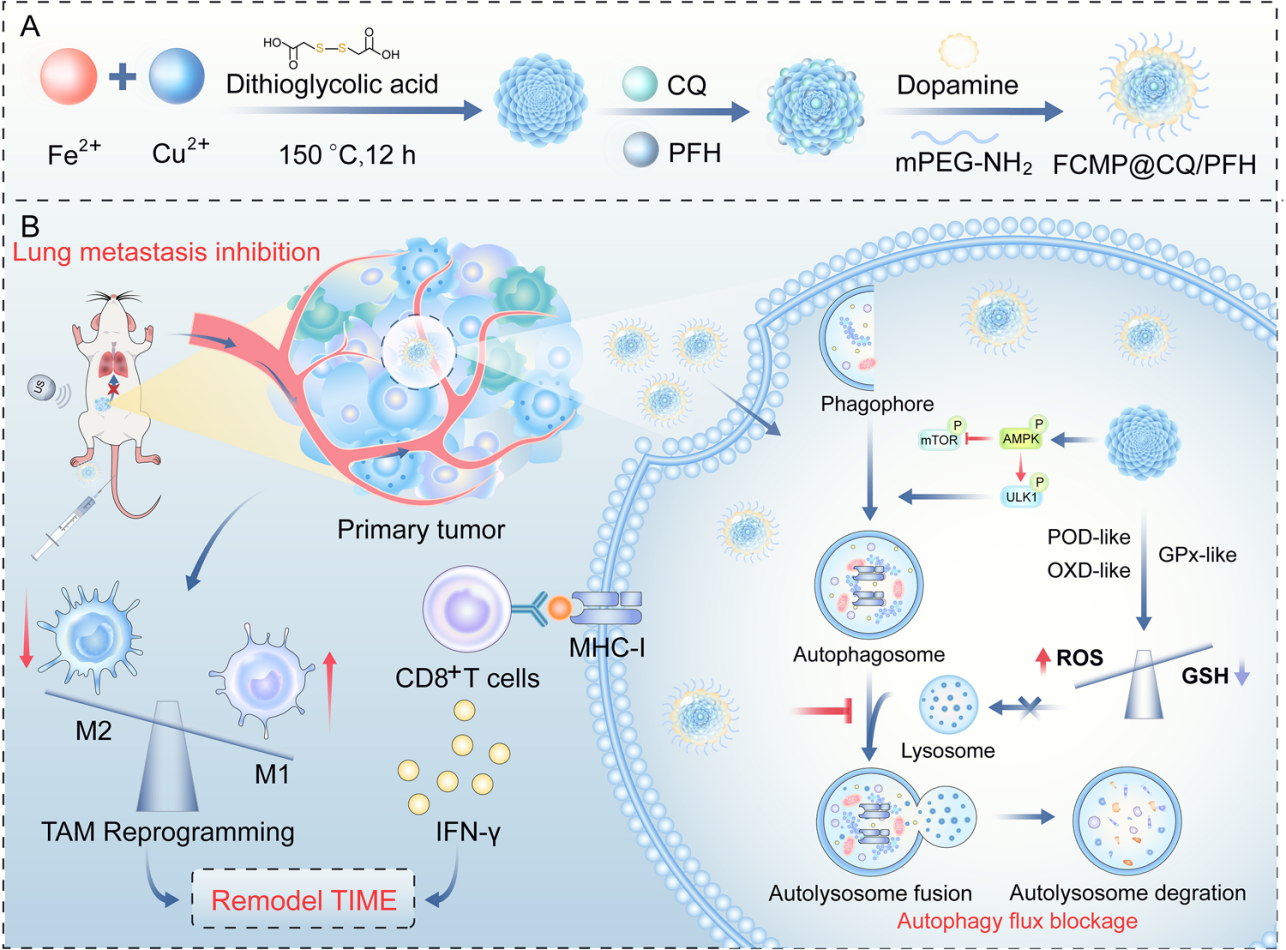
Ultrasound‐assisted FCMP@CQ/PFH nanoplatform with MOF‐based nanozyme activity for autophagy disruption and tumor immune microenvironment remodeling. A) Synthesis of FCMP@CQ/PFH. B) FCMP@CQ/PFH preferentially accumulates at the tumor site, where the MOF core generates ROS and depletes intracellular GSH via its intrinsic nanozyme‐like activity, inducing oxidative stress and disrupting autophagic flux by impairing autolysosome degradation. CQ further amplifies autophagy inhibition. These combined effects reprogram TAMs toward the M1 phenotype and enhance CD8⁺ T cell activation through upregulated MHC‐I expression and increased IFN‐γ secretion, thereby remodeling the tumor immune microenvironment and effectively suppressing tumor growth and metastasis.
